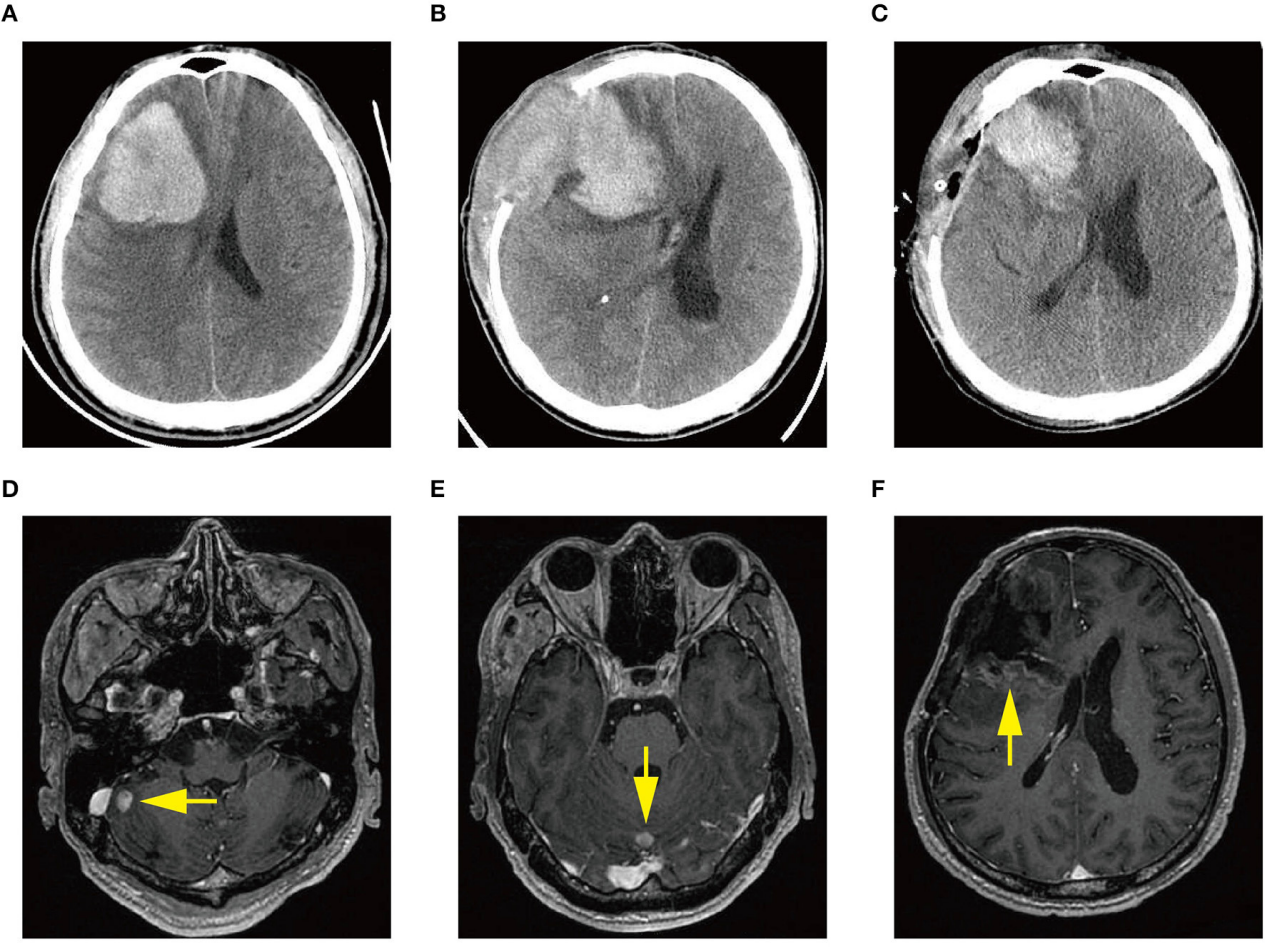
(A) Computed tomography (CT) scan at diagnosis demonstrating acute intracerebral hemorrhage (ICH) in the right frontal lobe. (B) CT scan demonstrating ICH re-occurrence at day 3 after the first craniotomy. (C) CT scan demonstrating rebleeding after the second craniotomy. (D–F) Magnetic resonance images showing multiple intracranial lesions (arrow) 1 month after the first craniotomy.
